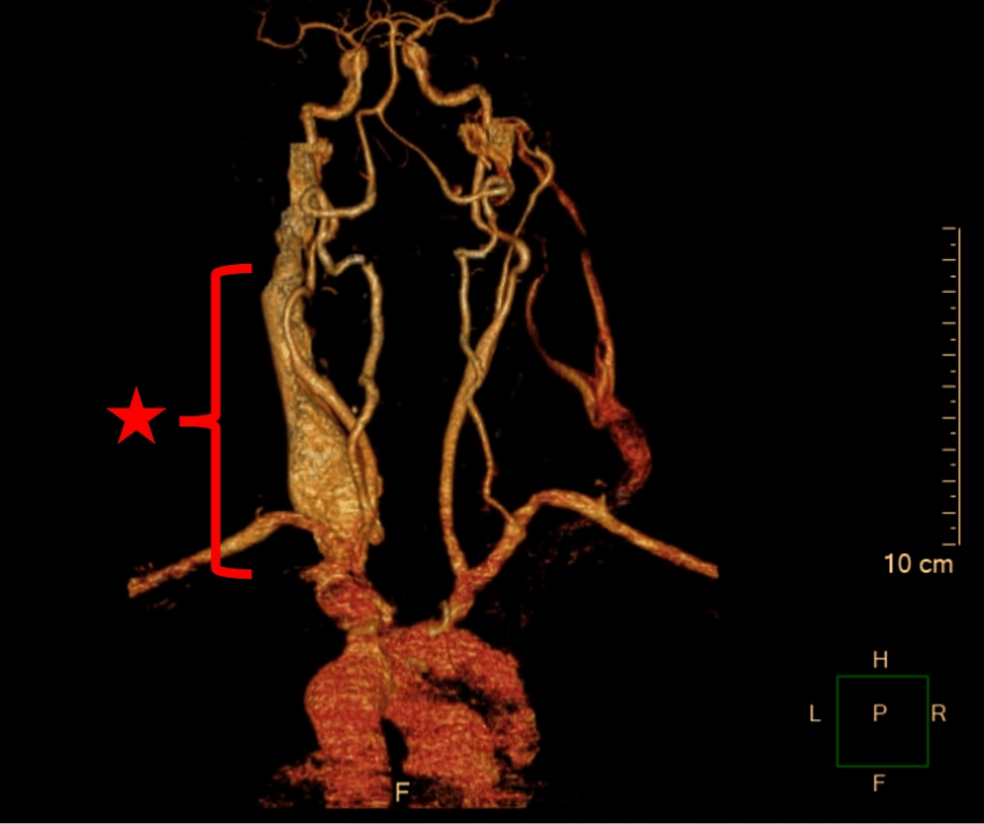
Computed tomography angiography of the neck showing irregular appearance of the right internal jugular in a 63-year old female This figure shows diffuse asymmetric enlargement and non-opacification of the right internal jugular vein (IJV) with adjacent soft tissue stranding (red bracket). Note that there is no appreciable right carotid stenosis or thrombosis, further excluding other diagnoses. These results are compatible with septic thrombophlebitis of the right IJV.
